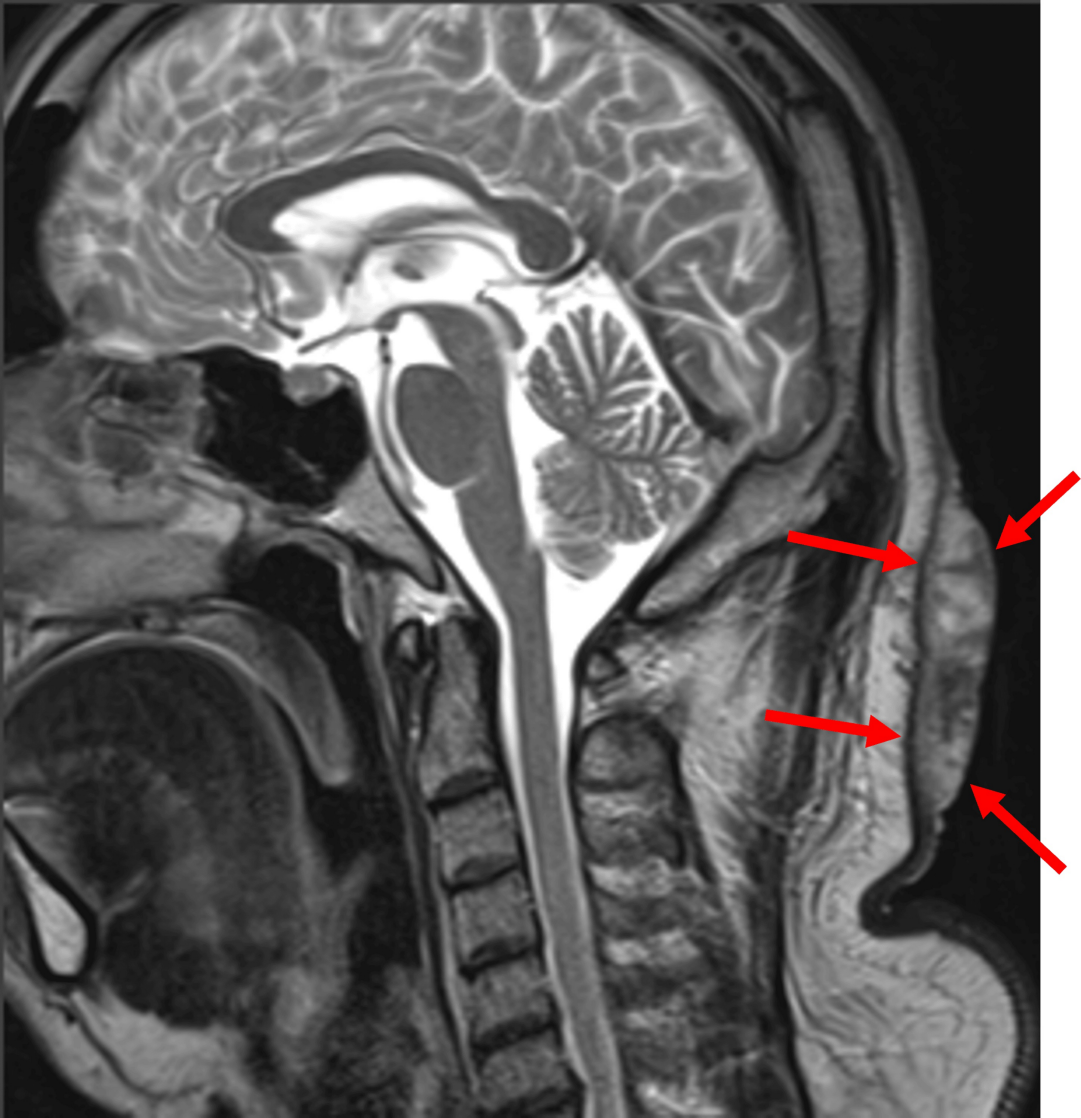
Preoperative magnetic resonance imaging (MRI) Sagittal view of the MRI
